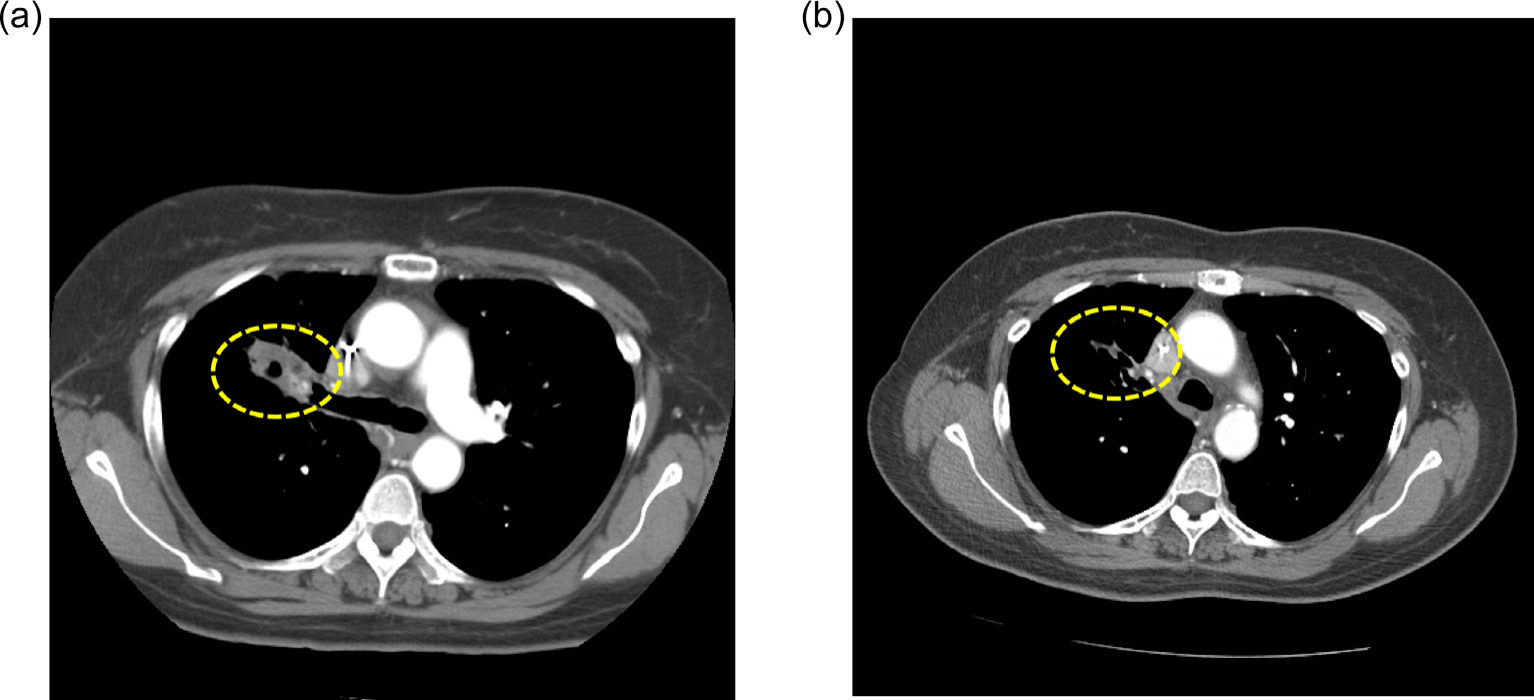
Computed tomography (CT) scans of a NSCLC patient (case #5, Table 2) with two sensitive *EGFR* mutations (L858R in exon 21 and G873E in exon 21) and a *PIK3CA* mutation (E542K in exon 9) a) CT at baseline, and b) CT taken 5 months after treatment initiation with erlotinib/cetuximab/bevacizumab demonstrating a PR (-55%). Duration of response = 9+ months. Patient had received prior erlotinib for 14.3 months.
